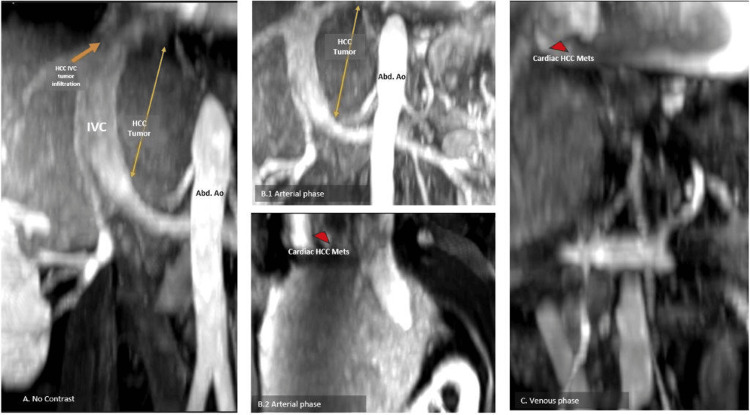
Triple-phase MRI of the liver with gadolinium enhancement demonstrating a hepatic mass with extensive tumor thrombus occluding essentially all the intrahepatic portal venous branches, supra-hepatic inferior vena cava, and right atrium, compatible with LR-TIV Liver MRI with intravenous contrast and a liver-specific protocol revealed an infiltrative liver mass occupying nearly the entire left lobe and parts of the central right lobe (A, B.1, and C). The mass extended as a tumor thrombus into the intrahepatic portal vein branches of both lobes, the main portal vein up to the portosplenic confluence, and further involved the left HV, extending to the suprahepatic IVC and the RA (B1 and 2). These imaging findings are consistent with HCC, classified as LI-RADS TIV. Additionally, a LI-RADS 4 lesion measuring 17 mm was identified in hepatic segment 5. The imaging also revealed sequelae of portal hypertension, including splenomegaly, a splenorenal shunt, a recanalized periumbilical vein, gastric varices, and small-volume ascites. MRI: magnetic resonance imaging; IVC: inferior vena cava; RA: right atrium; HV: hepatic vein; HCC: hepatocellular carcinoma; LI-RADS TIV: liver imaging reporting and data system tumor in vein
